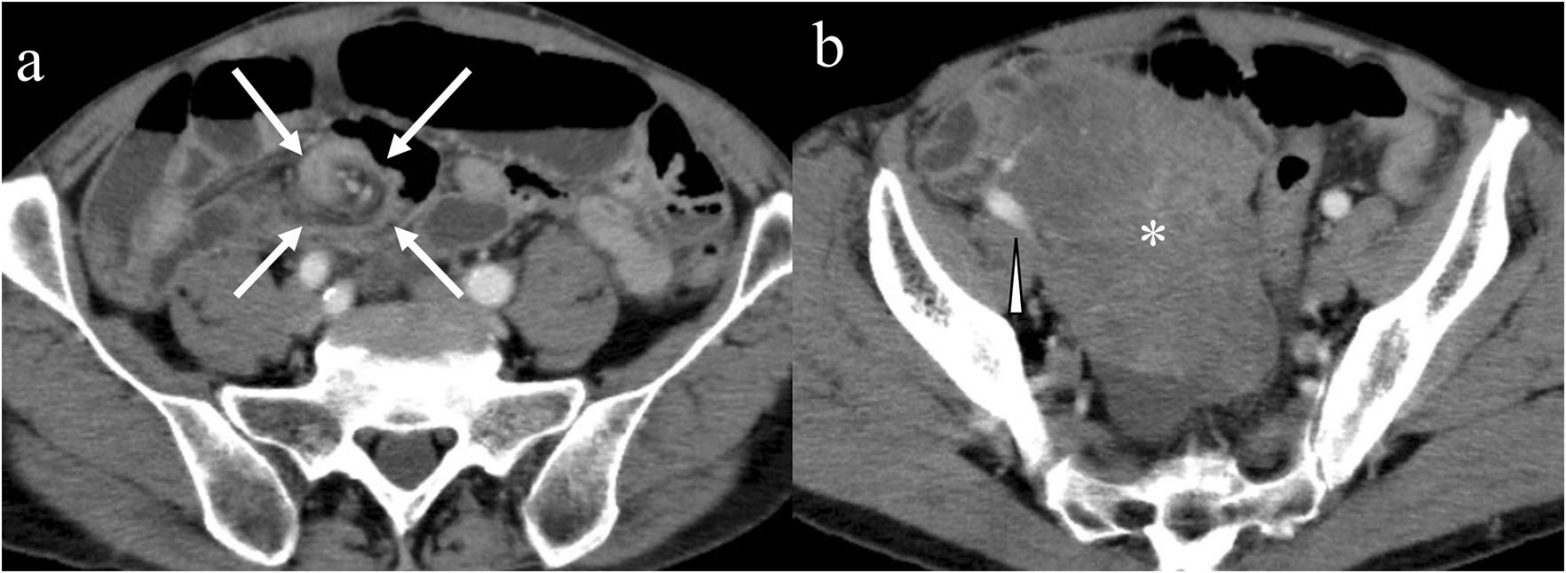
Mesenteric volvulus caused by an exophytic gastrointestinal stromal tumor in the small intestine. A 74-year-old male presented with abdominal discomfort and bloating. Contrast-enhanced CT shows the whirl sign in the mesentery, but enhancement is preserved in the involved mesenteric vessels (a: arrows). A > 10 cm tumor is seen in the pelvis (b: asterisk). The dilated drainage vein with enhancement is observed adjacent to the tumor (b: arrowhead). Laparotomy showing a large exophytic lobulated tumor arising from the small bowel on the antimesenteric side
